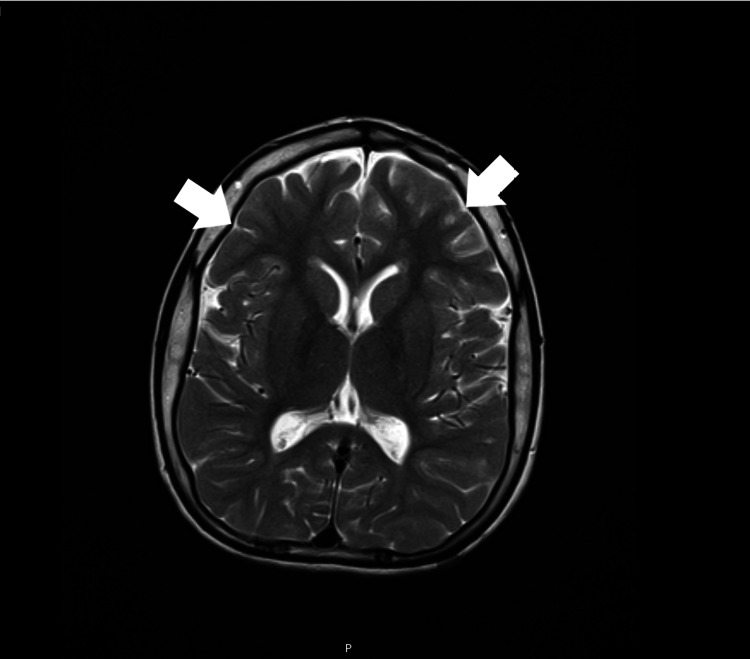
Patient 1's brain MRI, performed at the age of 13. Seen are diffuse bilateral cortico-subcortical moderate atrophy, mainly symmetrical; slight frontotemporal predominance (arrows); and thickening of the cranial vault.
